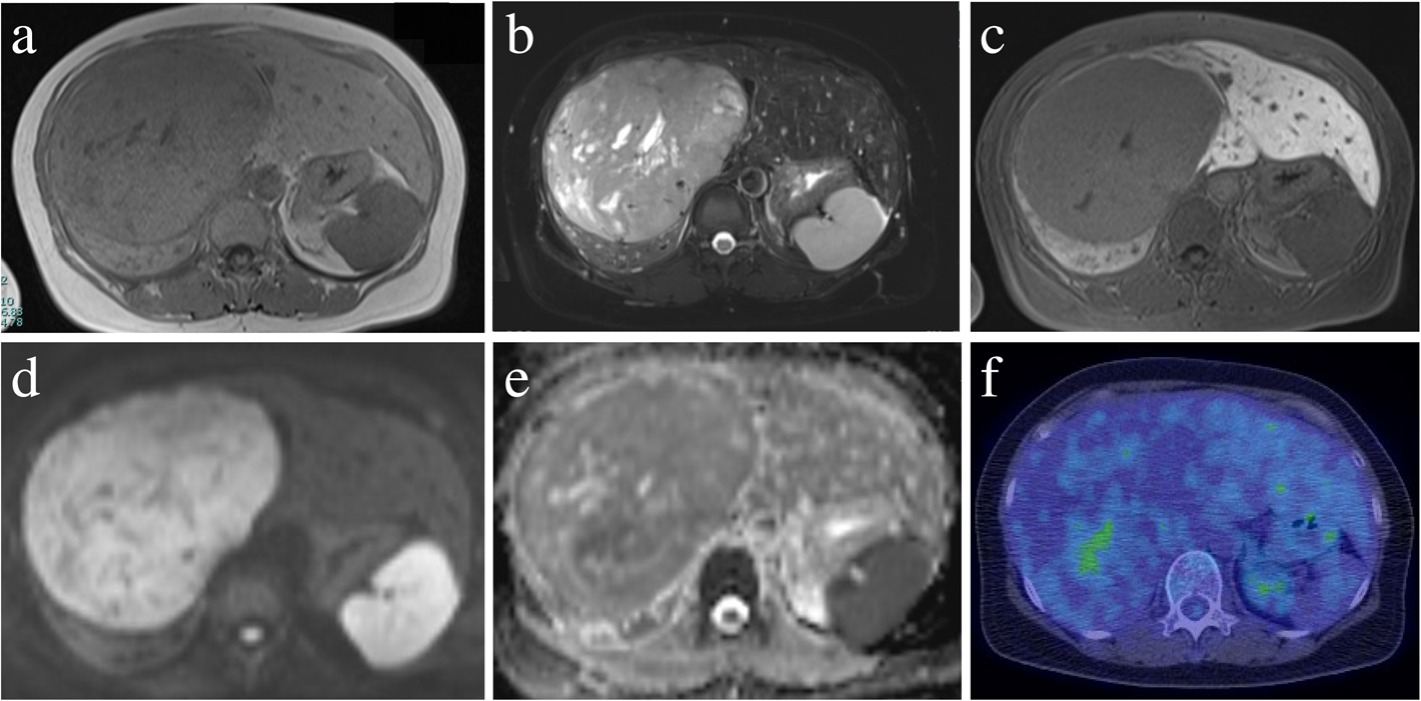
Magnetic resonance imaging (MRI) and [^18^F]-fluorodeoxyglucose positron emission tomography (FDG-PET). Abdominal MRI shows low intensity on a T1-weighted image (a) and heterogeneously high or iso intensity on a T2-weighted image (b). Gadolinium-ethoxybenzyl-diethylenetriamine pentaacetic acid-enhanced MRI reveals a hypointense mass during the hepatobiliary phase (c). Diffusion-weighted imaging shows higher intensity than for normal liver parenchyma (d) with a high *b* value of 1000 (e). FDG-PET shows no accumulation of [^18^F]-FDG (f)
